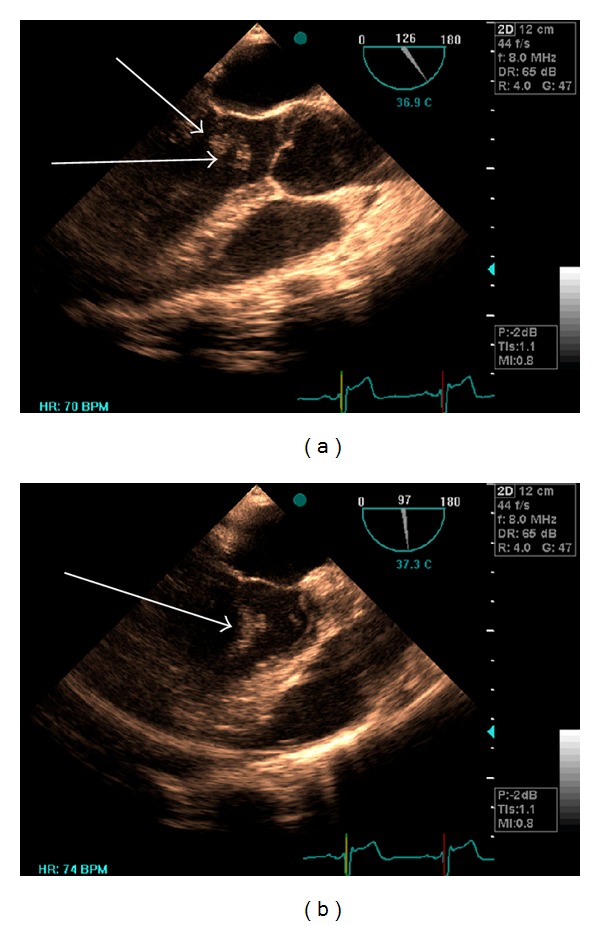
Transesophageal echocardiographic image showing mobile LV masses attached to the mitral valve and the posterior wall extending to LV outflow tract.
